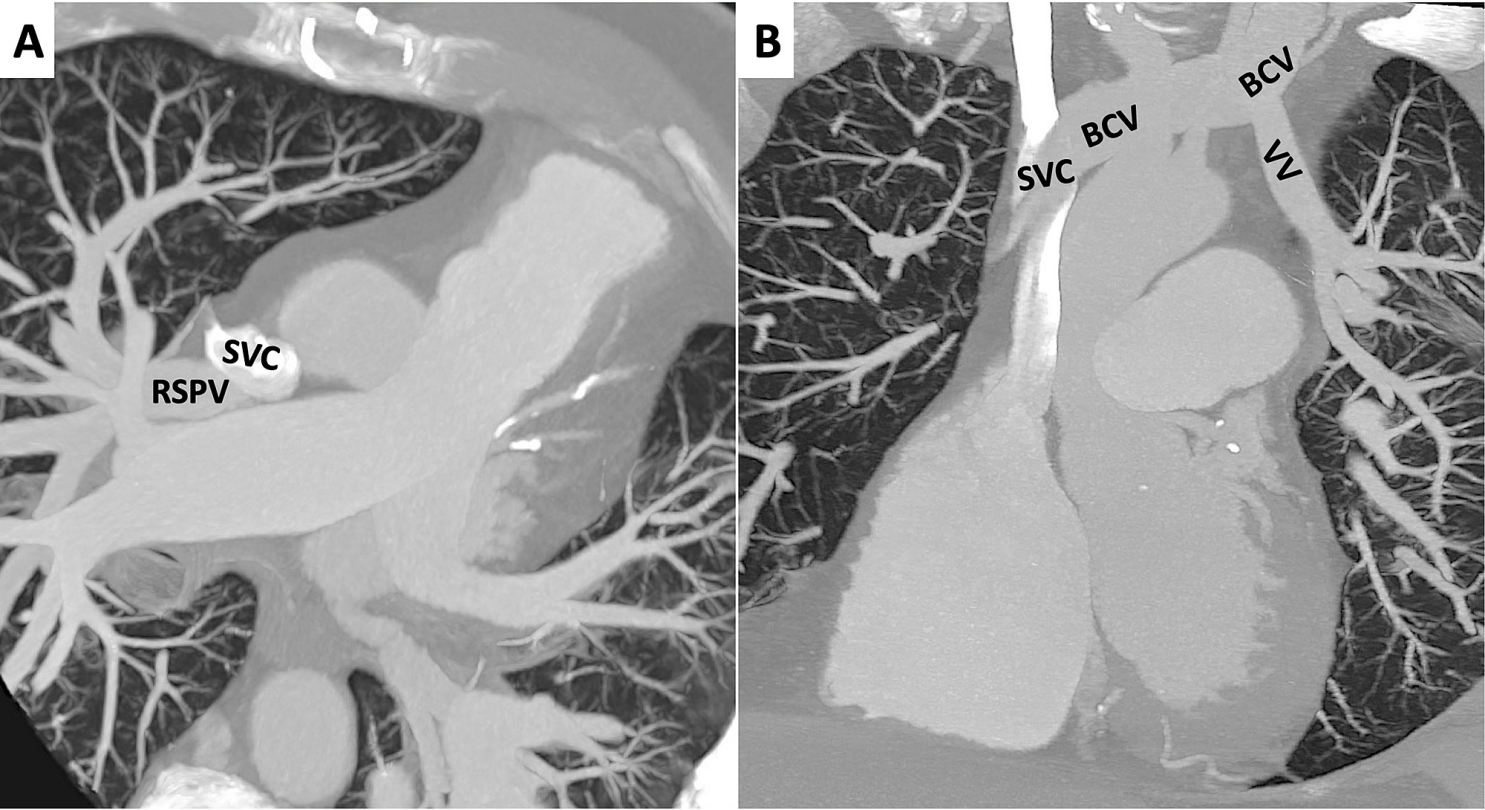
Cardiac computed tomography: Anomalous venous return (A) Maximum Intensity Projection (MIP) images in axial plane. Right superior pulmonary vein (RSPV) drains into the superior vena cava (SVC). (B) MIP images in coronal plane. Left superior pulmonary vein drains into the brachiocephalic vein (BCV) through a vertical vein (VV) and the latter drains into the SVC.
